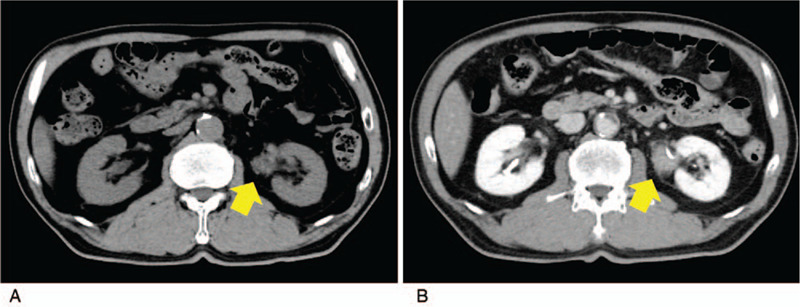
Abdominal computed tomography images on admission. (A: image without contrast enhancement; B: image with contrast enhancement). The images reveal left renal pelvis thickening of the retroperitoneum with encasement of the ureters (arrows).
